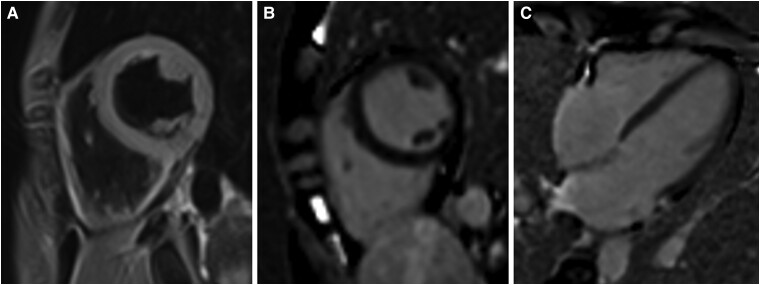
T2-weighted and gadolinium-enhanced cardiac magnetic resonance imaging. (*A*) Short axis view of T2-weighted short-tau inversion recovery imaging. Regional high signal intensities or increased global signal intensity ratio was not identified in the myocardium. (*B*) Short axis and (*C*) Four-chamber view of gadolinium-enhanced imaging. Delayed contrast was not identified in the myocardium.
